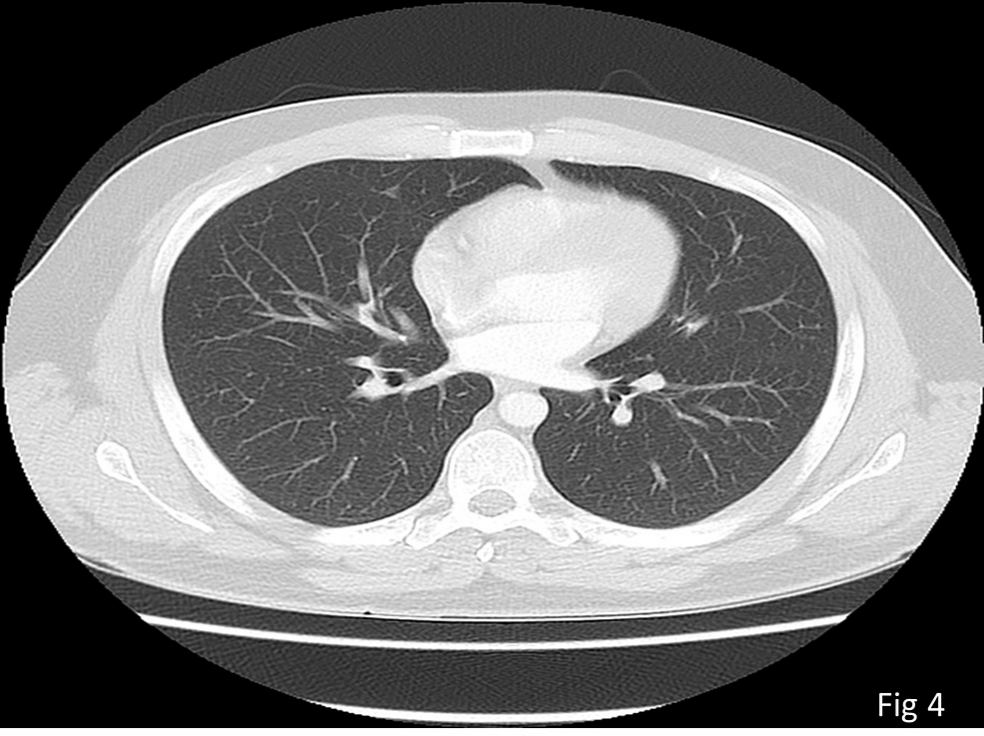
CT image of a healthy lung CT image of healthy lungs showing normal CT ratio. Normal bronchovascular markings can be visualized. The image is adapted from radiopedia.org (free access). Image source: Reference no. [12]
